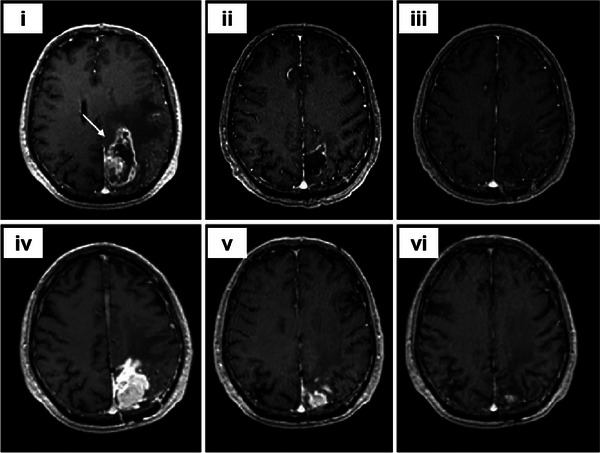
Axial T1‐weighted MRI scans were taken after fractionated stereotactic radiotherapy (FSRT) and temozolomide treatment for recurrent GBM: The arrow points to a large enhancing mass in the parietal lobe (i). Three weeks post‐surgery, the patient underwent radiotherapy and temozolomide treatment (ii). Post‐treatment imaging showed significant changes in the tumor (iii). After 20 months, the tumor recurred and was treated with FSRT and temozolomide (iv). Post‐treatment MRI scans at 2 months (v) and 6 months showed gradual tumor shrinkage (vi). Reproduced with permission.^[^
